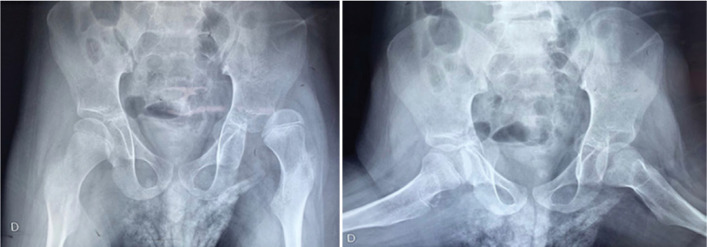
Preoperative pelvic radiographs in the AP and frog-leg views (left and right, respectively) of a child with cerebral palsy and left hip dislocation.
